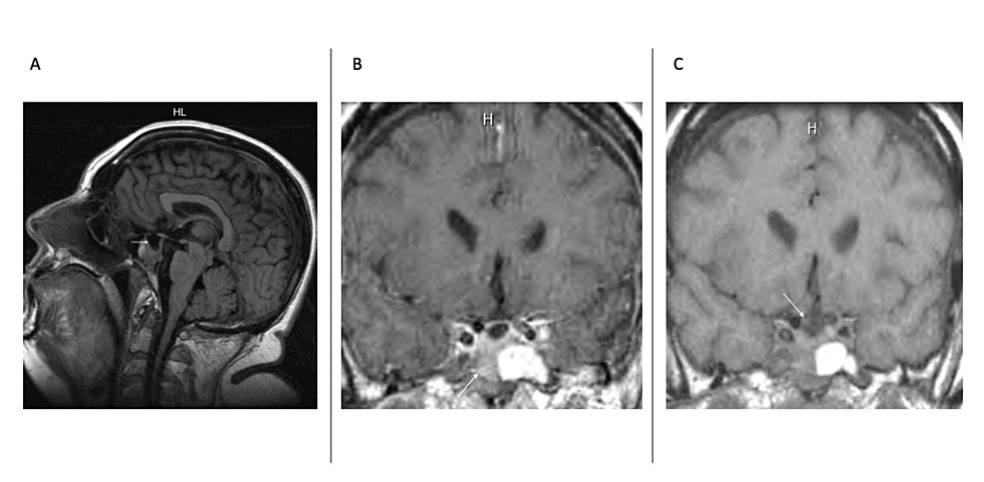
MRI of the sella turcica showing an empty sella (A), a mass in the right posterior sphenoid sinus (B), and no evidence of a sellar mass (C)
